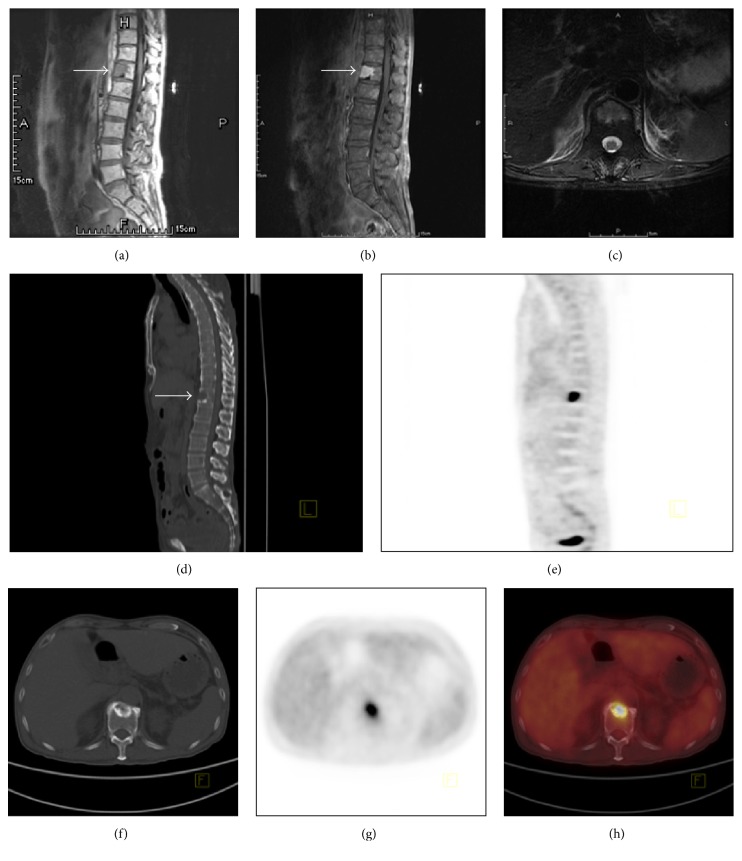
Images of the bone lesion detected by MRI and (18) F-FDG PET/CT. (a)~(c): Short-tau inversion-recovery (STIR) sequence in MRI shows a bone lesion in T12 vertebra, with T1-hypointense and T2-hyperintense signal. (a) Sagittal TI STIR image. (b) Sagittal T2 STIR image. (c) Transaxial T2 STIR image. (d)~(h): (18) F-FDG PET/CT image shows an osteosclerotic lesion, with hypermetabolism in T12 vertebra. (d) Sagittal CT image. (e) Sagittal PET image. (f) Transaxial CT image with a significant sclerotic ring around the bone lesion. (g) Transaxial PET image with an average SUV value of 12.4. (h) Fused PET/CT image.
